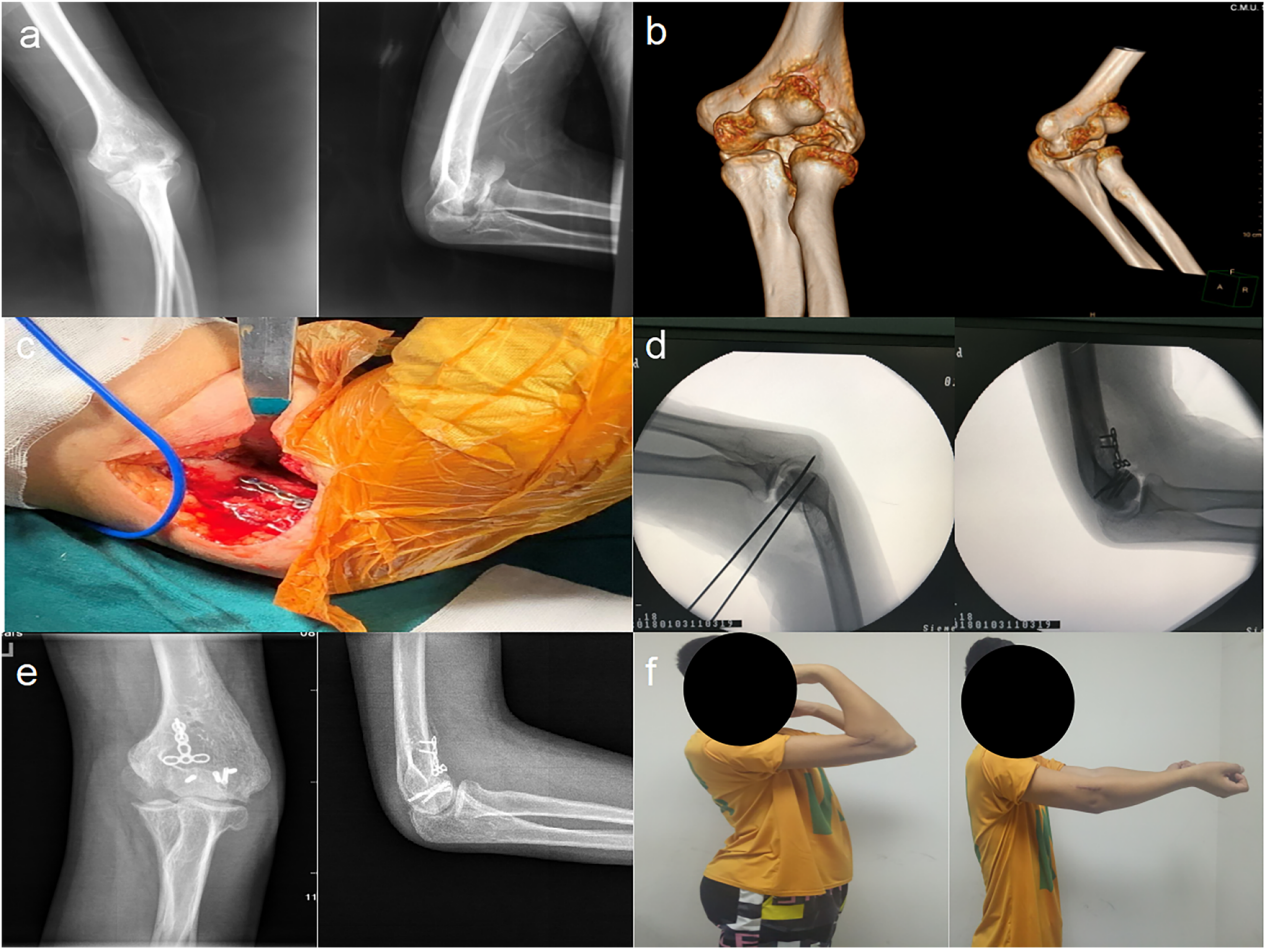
Dubberley type 3A fracture: a A 24-year-old male had a coronal shear fracture of distal humerus (Dubberley 3A) with preoperative X-ray images of distal humerus. b Preoperative CT images showed seperated fragments of capitellum and trochlea. c Extended lateral provided an optimal window to exposure and a mini T-shaped plate was used to anti-resist the coronal shear forces of distal humerus. d Intraoperative images of C-arm guided fracture reduction and internal fixatoin. e Postoperative X-ray images showed good internal fixation with three headless compression screws and a mini T-shape plate for fracture fixation. f At final following-up the young man had good range of motion and returned to former work.
